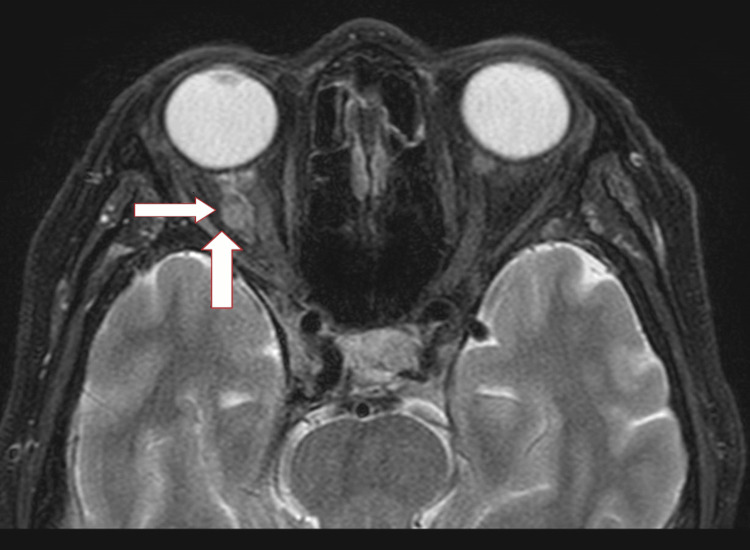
MRI orbits show a well-defined intraconal lesion measuring 0.5 x 0.5 x 0.5 cm (AP X W X CC) at the lateral aspect of the right optic nerve in the intraorbital segment. The lesion was displacing the right optic nerve medially.
